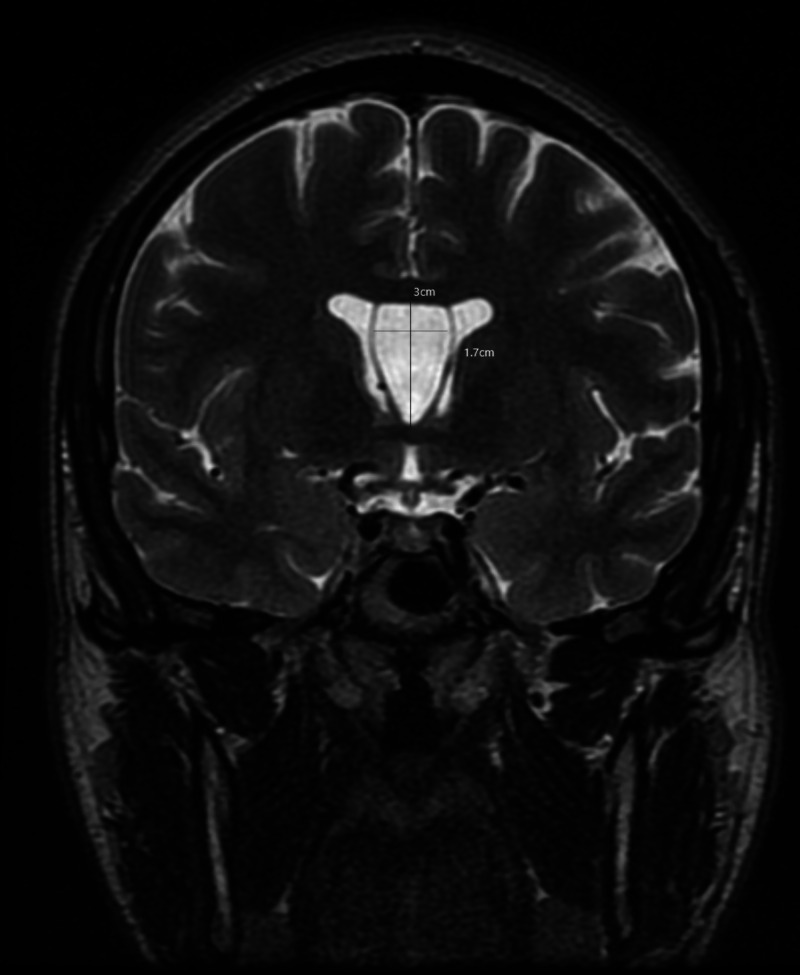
T2 weighted magnetic resonance imaging (MRI) of the head in coronal view This is a T2 weighted magnetic resonance imaging (MRI) of the head in coronal view that is showing the full longitudinal extent of the cyst of the cavum septum pellucidum et vergae. Lateral bowing of the walls of the cavum is clearly visible which is one of the criteria required to diagnose a cyst of the cavum septum pellucidum.
